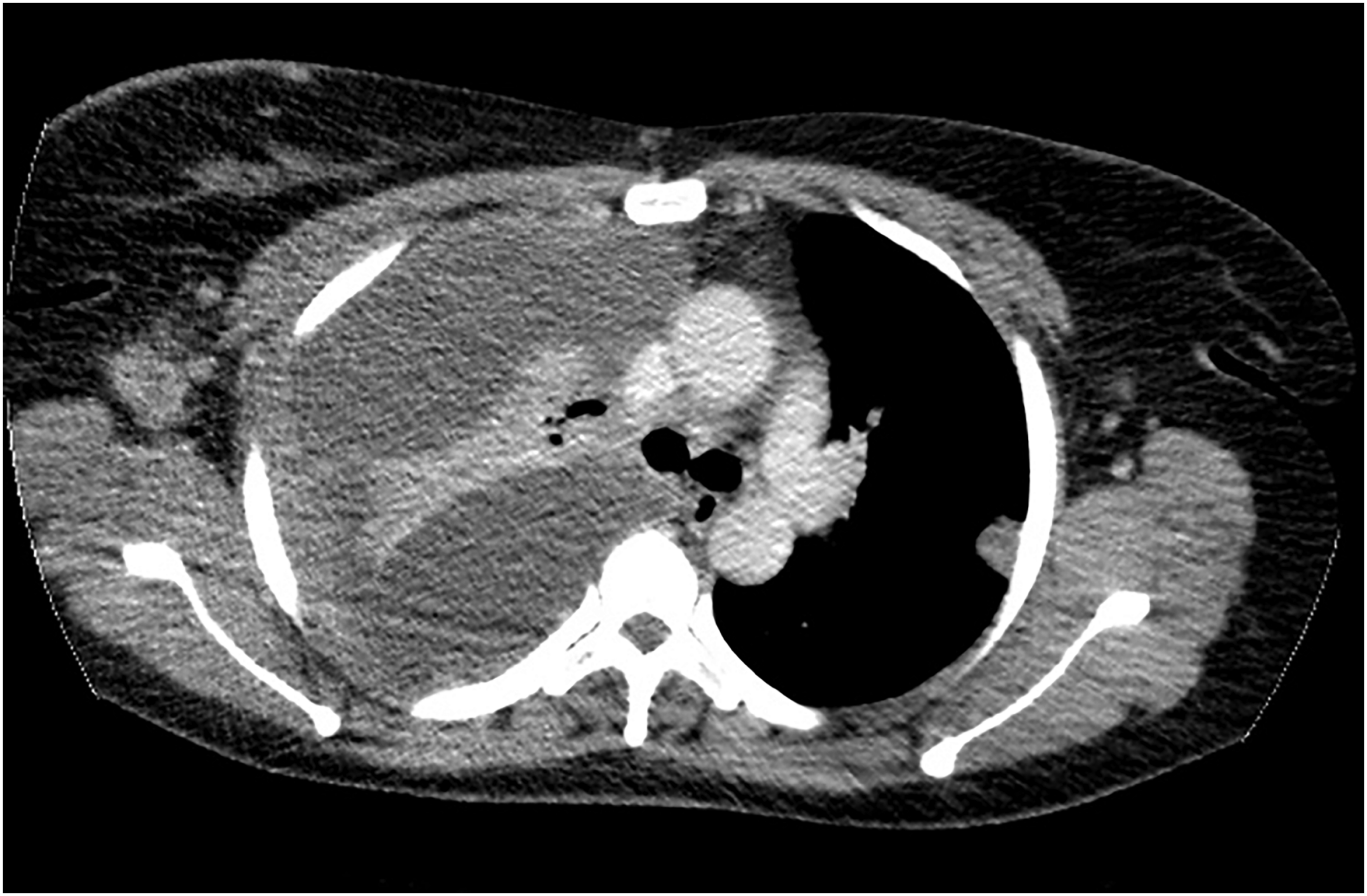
Axial computed tomography scan image of the chest, mediastinal window showing massive right pleural effusion, pleural thickening and left pleural nodule, highly suggestive of metastatic disease.
